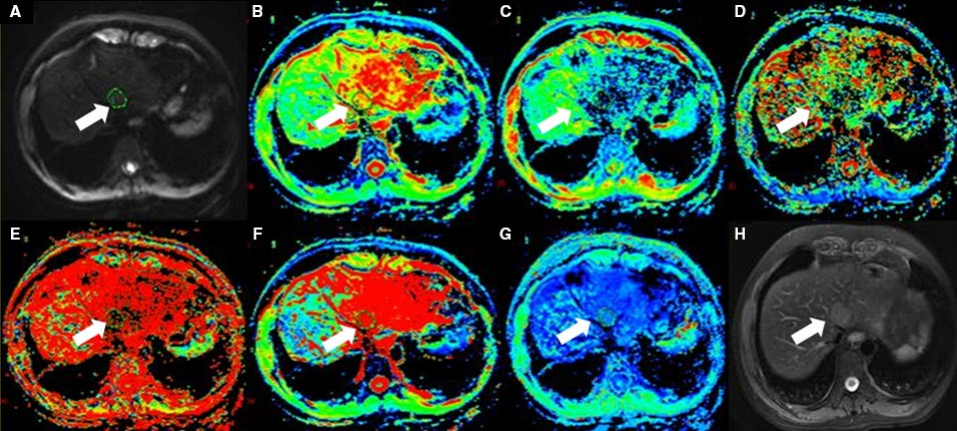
Thirty‐two‐year‐old male with hepatic inflammatory nodules(arrow). (A) is b value of 50 s/mm^2^ of DWI, and (B‐G) are pseudocolor of ADC,* D*
